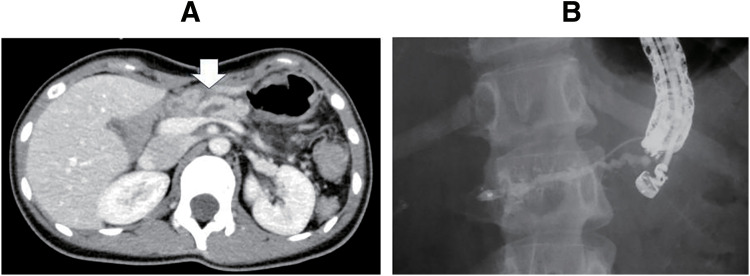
Preoperative imaging findings. The patient experienced repeated abdominal pain due to pancreatitis after LPD for solid pseudopapillary neoplasm of the pancreatic head. A CT scan revealed a dilated main pancreatic duct, indicated by the arrow (A). Since the anastomosis could not be identified by endoscopy, the main pancreatic duct was punctured under endoscopic ultrasound (B). The guidewire could not pass through the anastomosis, and an endoscopic nasal pancreatic drainage tube was placed in the main pancreatic duct. LPD, laparoscopic pancreaticoduodenectomy
